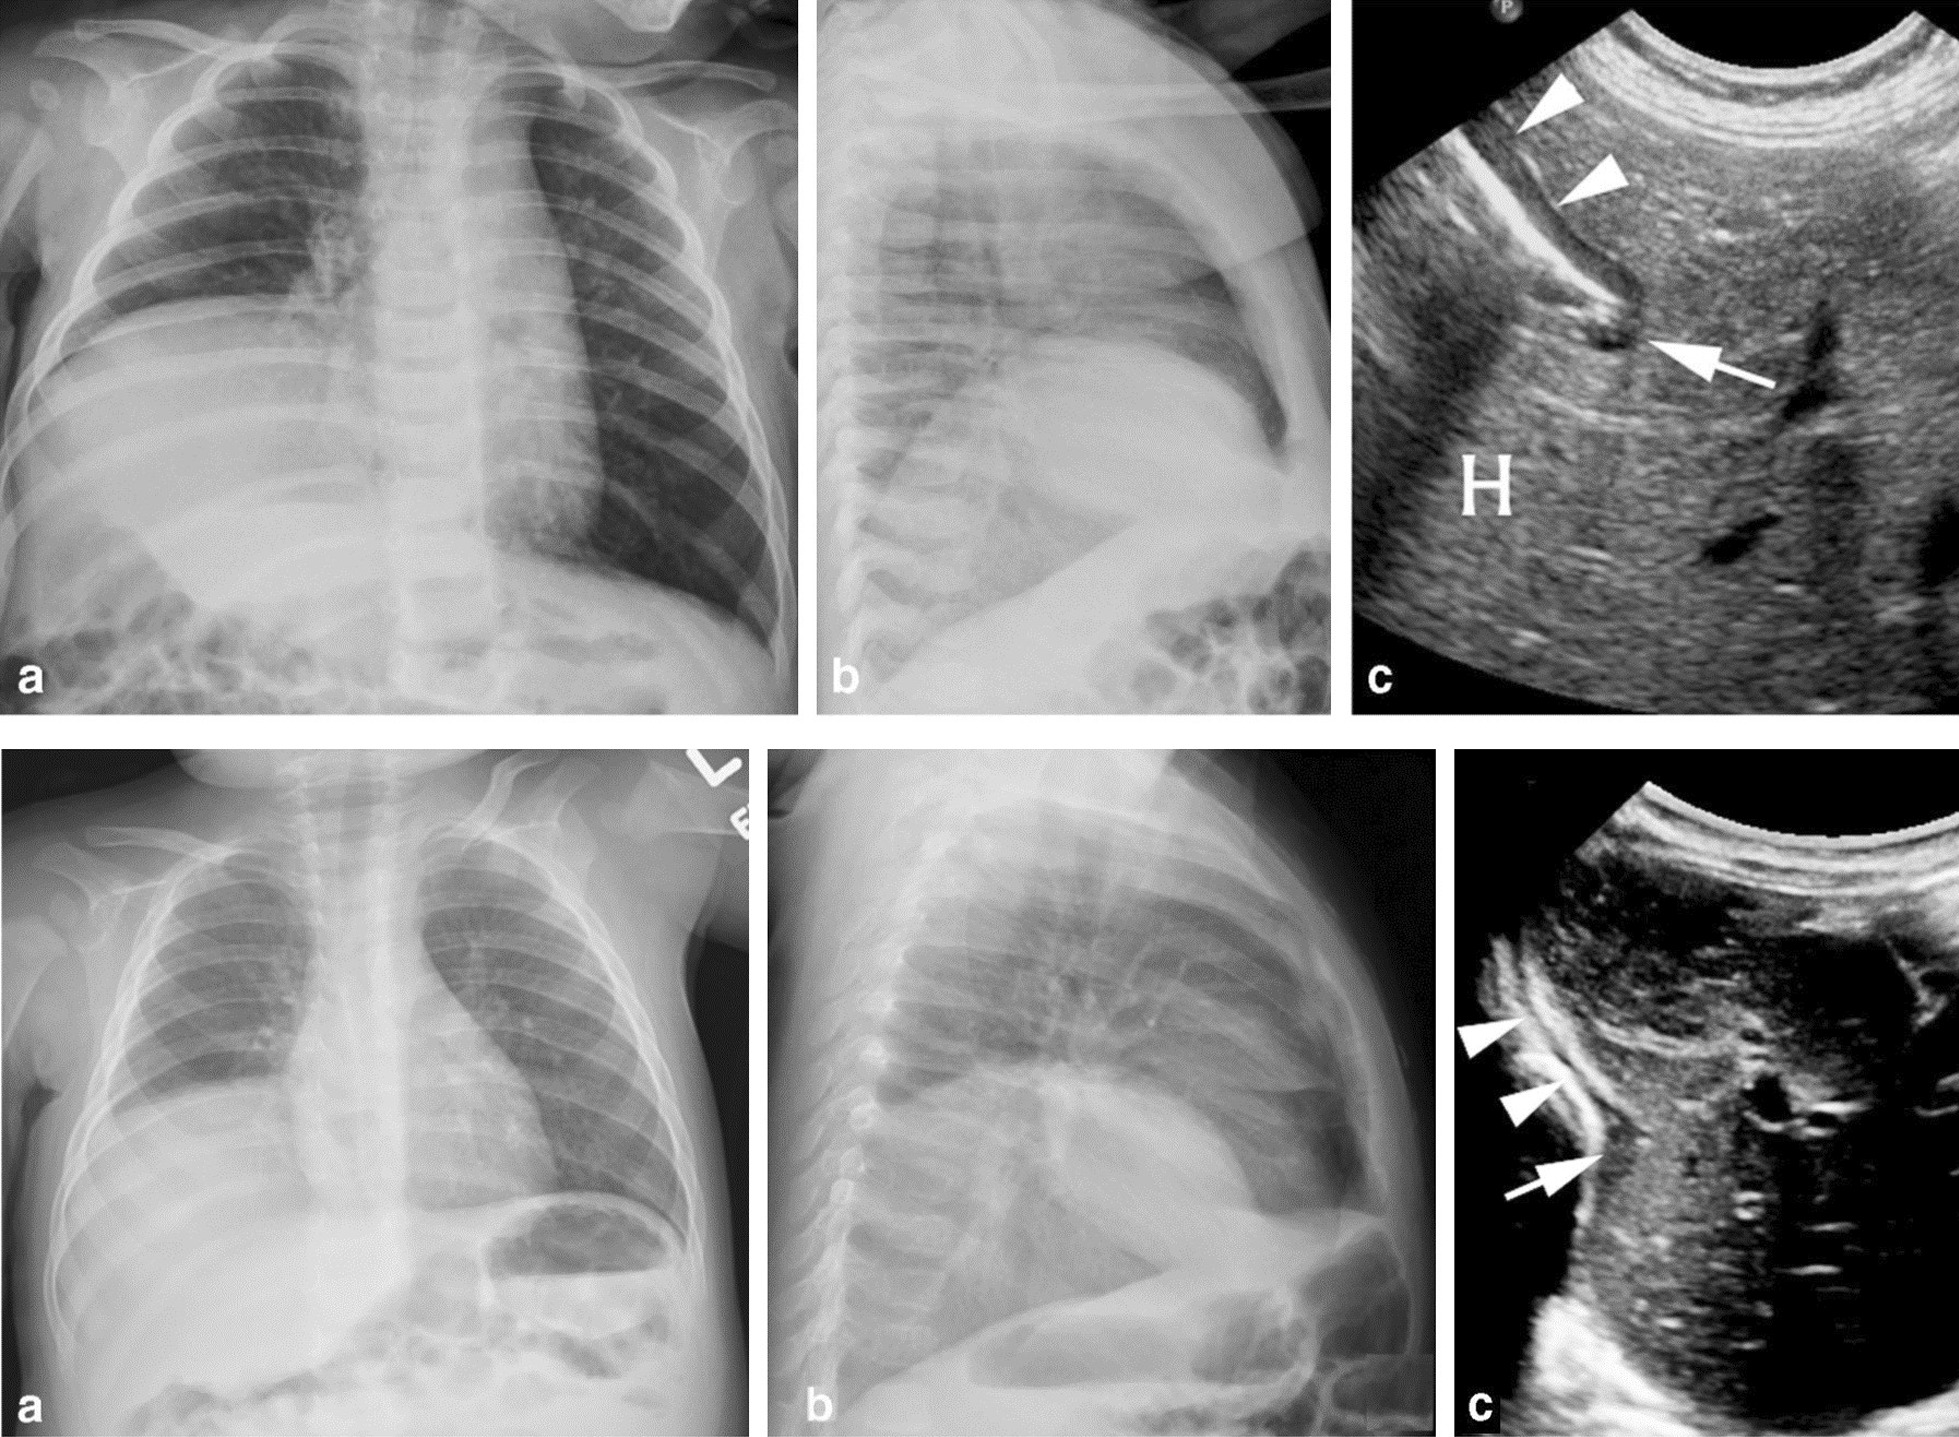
Chest radiographs and ultrasound images in representative patients with diaphragmatic hernia *(upper panels)* and diaphragmatic eventration *(lower panels). (Upper panels)* A 5-month-old boy, with right Bochdalek hernia. Anteroposterior and lateral chest radiographs demonstrate what would appear as global elevation of the right hemidiaphragm (a, b). Lateral long-view ultrasound (c) demonstrates the normal anterior hypoechogenic muscle (arrowheads), the folding free edge of the diaphragm at a narrow angle waist (arrow), and the herniated liver through the diaphragmatic defect (H). *(Lower panels)* A 9-month-old boy, with right diaphragmatic eventration. Anteroposterior and lateral chest radiographs demonstrate global elevation of the right hemidiaphragm (a, b). Long-view ultrasound (c) demonstrates posterior elevation of the diaphragm with a wide-angle waist. The normal anterior hypoechogenic diaphragm (arrowheads) with focal thickening of the muscle (arrow) covering the entire waist. Reproduced with permission from Springer Nature: Karmazyn et al. Pediatr Radiol 2019;49(8):1010–7
